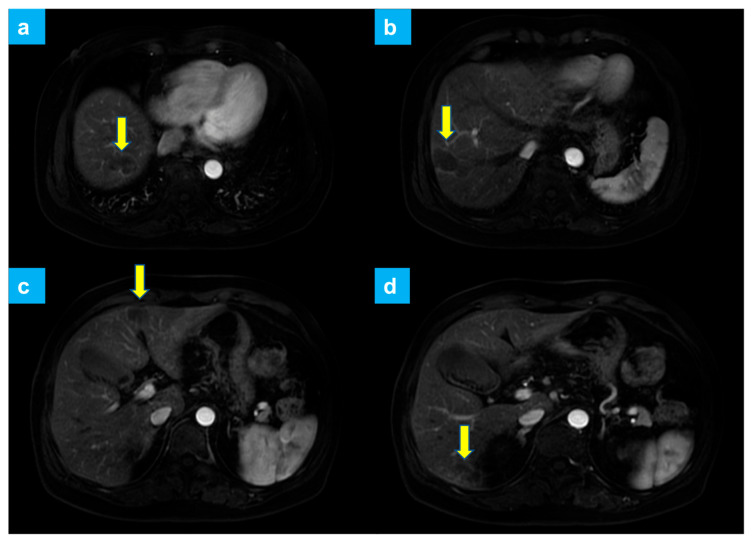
The case shown in Figure 2. Two months after TA, the enhanced MRI showed no obvious enhancement on the scan (yellow arrow). (a–d) show the imaging changes of liver metastases in four sites of the patient shown in Figure 2 after TA.
